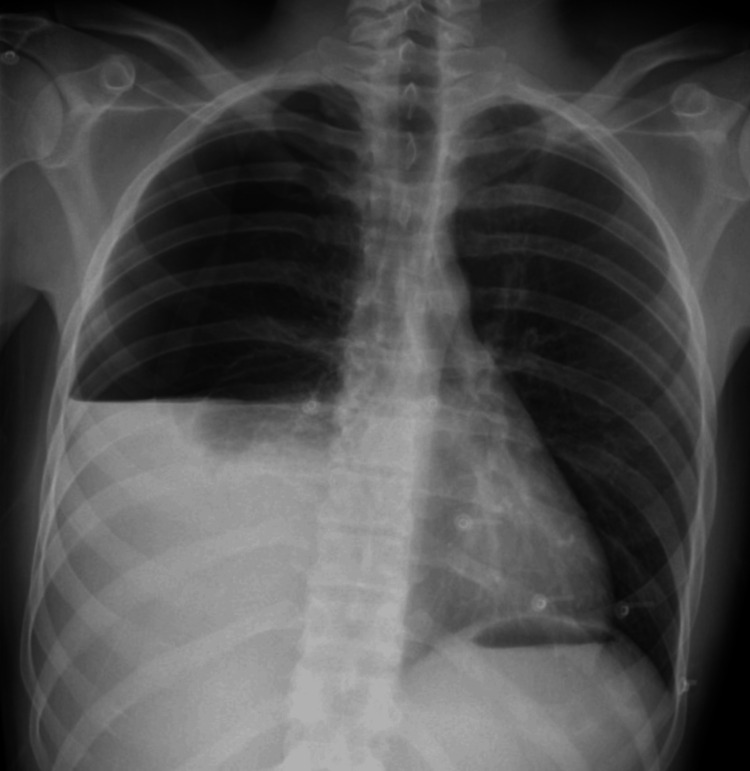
Chest X-ray demonstrating the hemothorax
